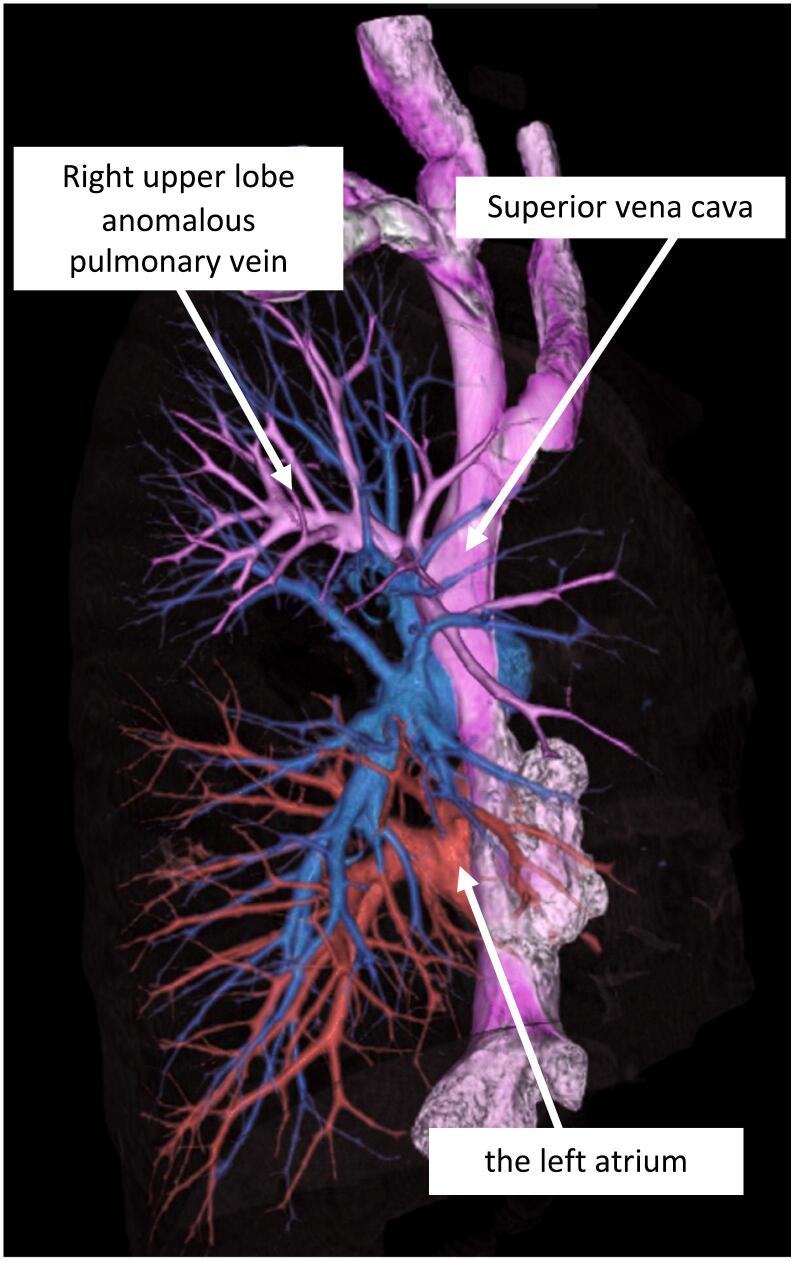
Three-dimensional computed tomography. A 3D computed tomography image of the pulmonary vessels showed the absence of a right upper lobe pulmonary vein draining into the left atrium, and a partial anomalous pulmonary venous connection consisting of drainage of the right upper lobe pulmonary vein into the superior vena cava.
